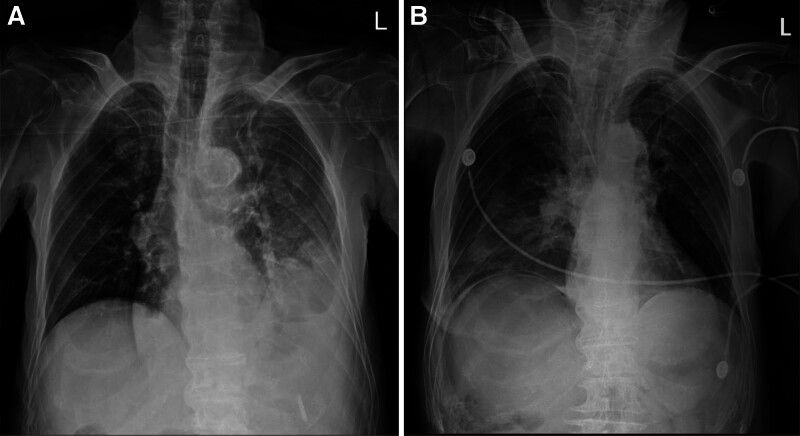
(A) Chest X-ray revealing a bowel shadow in the left thoracic cavity, indicating hiatal hernia. (B) Resolution of the lesion immediately after surgery.
